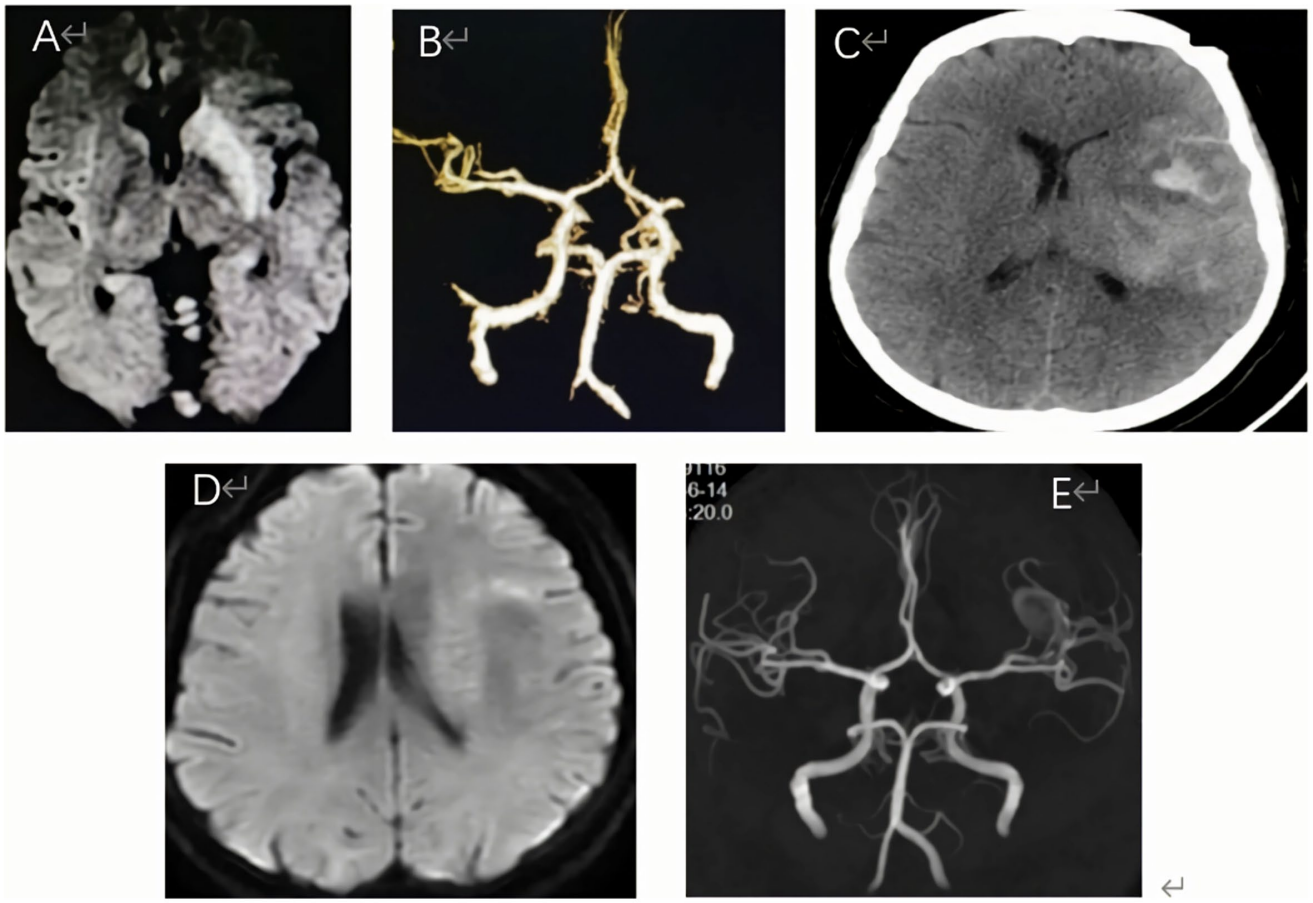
(A) Diffusion-weighted imaging (DWI) demonstrated prominent hyperintense signals within the basal ganglia and caudate nucleus, indicating potential acute ischemic changes. (B) Magnetic resonance angiography (MRA) confirmed the presence of an occlusion in the left middle cerebral artery. (C) A non-contrast CT head scan found contrast extravasation, and no intracranial hemorrhage or hyperperfusion. (D) Three days after oneset, brain MRI showed significant improvement in the high signal on the basal ganglia, but no significant increase of infarcted tissue. (E) Brain MRA indicated complete recanalization of the left MCA on the third day after operation.
